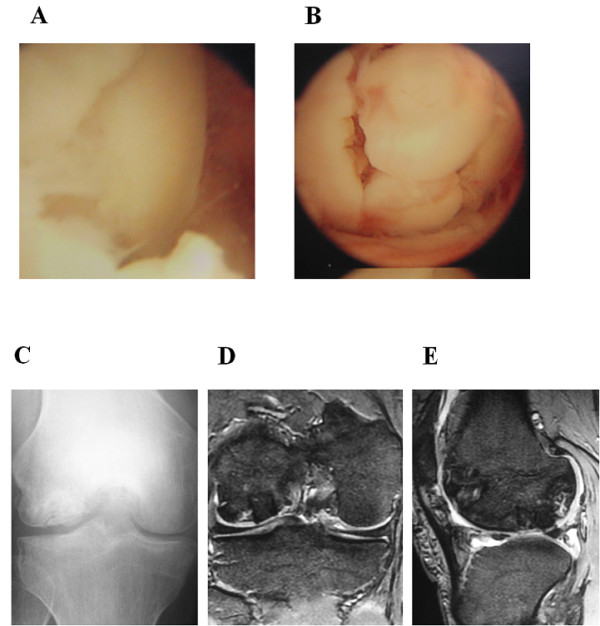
Operative and postoperative findings in case 1. (A) Arthroscopy showing the lesion of the osteonecrosis at the medial condyle of the femur demonstrating the anterior margin of the femoral condylar defect. (B) Osteochondral plugs are grafted into the recipient site. (C) Anteroposterior radiograph taken one year after surgery shows less irregularity of the subchondral bone of the lateral femoral condyle and a larger sclerotic area. (D, E) Coronal and saggital MRIs taken one year after surgery also show less irregularity of the subchondral bone and the reduction of the osteonecrotic area as compared with the preoperative MR images (Figure 1B,C), although a high signal area remains slightly in the lateral and posterior aspect of the lesion.
